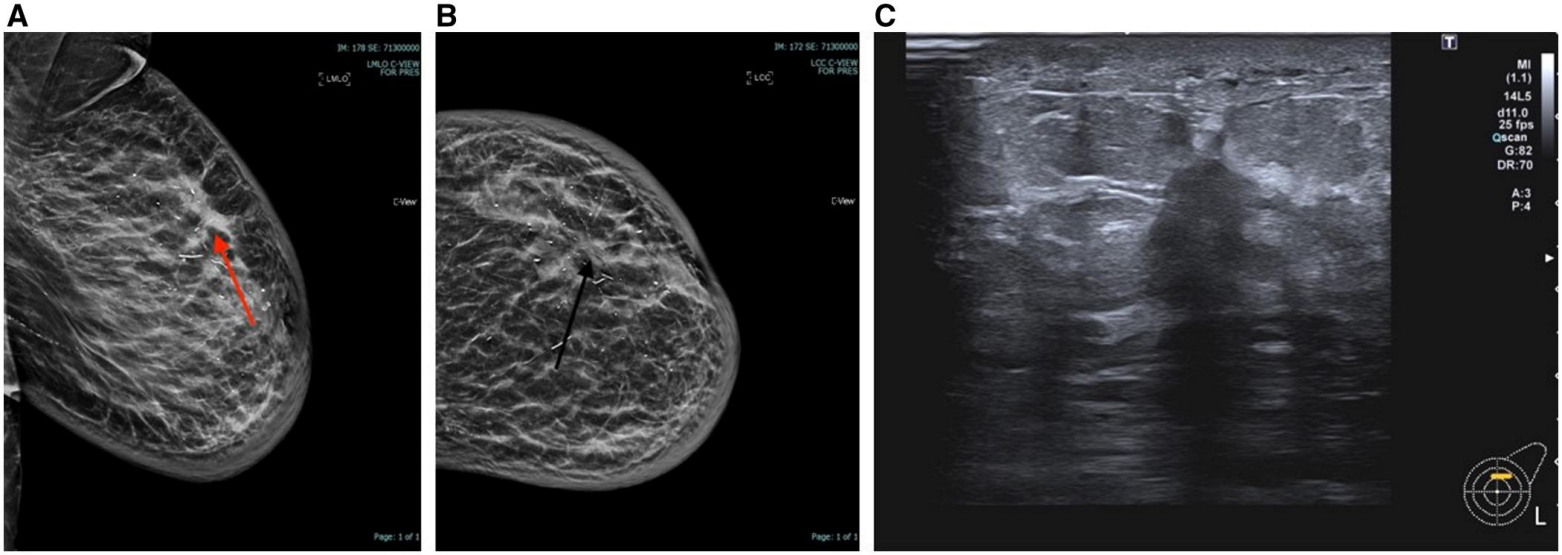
(A) MLO View showing distortion, (B) CC view showing distortion, (C) US image showing sonographic correlate corresponding to distortion. US-guided biopsy with malignant results (IDC grade 2).
